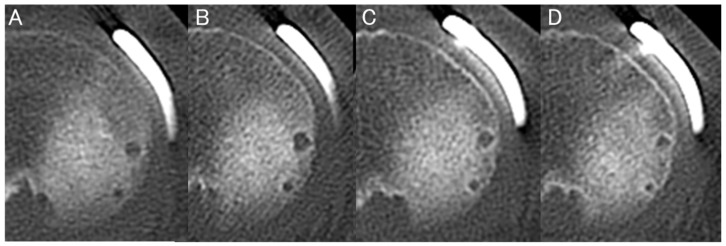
Representative serial changes in AHW observed on MPR CT images at 1 month (A), 3 months (B), 6 months (C), and 1 year (D) postoperatively. AHW increased and peaked at approximately 3 months, then gradually decreased with surrounding bone sclerosis by 6 months to 1 year postoperatively.
